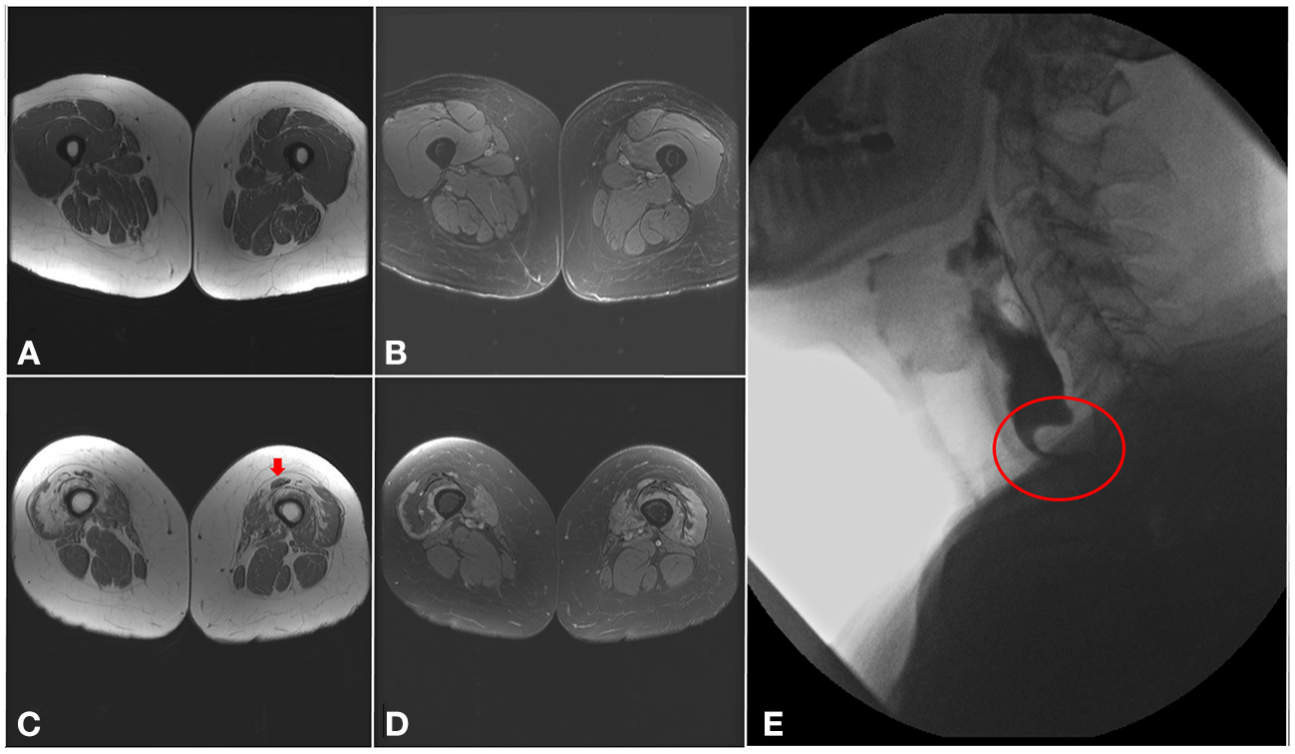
Imaging findings in inclusion body myositis. (A–D) MRI of the thighs from a patient with IBM. Axial T1 images (A,C) showing a proximal-to-distal gradient, with preservation of proximal segments (A) and fatty infiltration of the distal vastus medialis and lateralis bilaterally (C), more pronounced in the right lower limb, with relative sparing of the rectus femoris (arrow). Axial T2 images (B,D) demonstrating sparing of the proximal segments of the quadriceps muscles (B), and only mild T2 hyperintensity surrounding areas of fatty infiltration distally (D). (E) Barium swallow demonstrating a cricopharyngeal bar with severe (more than 75%) luminal narrowing (circle).
